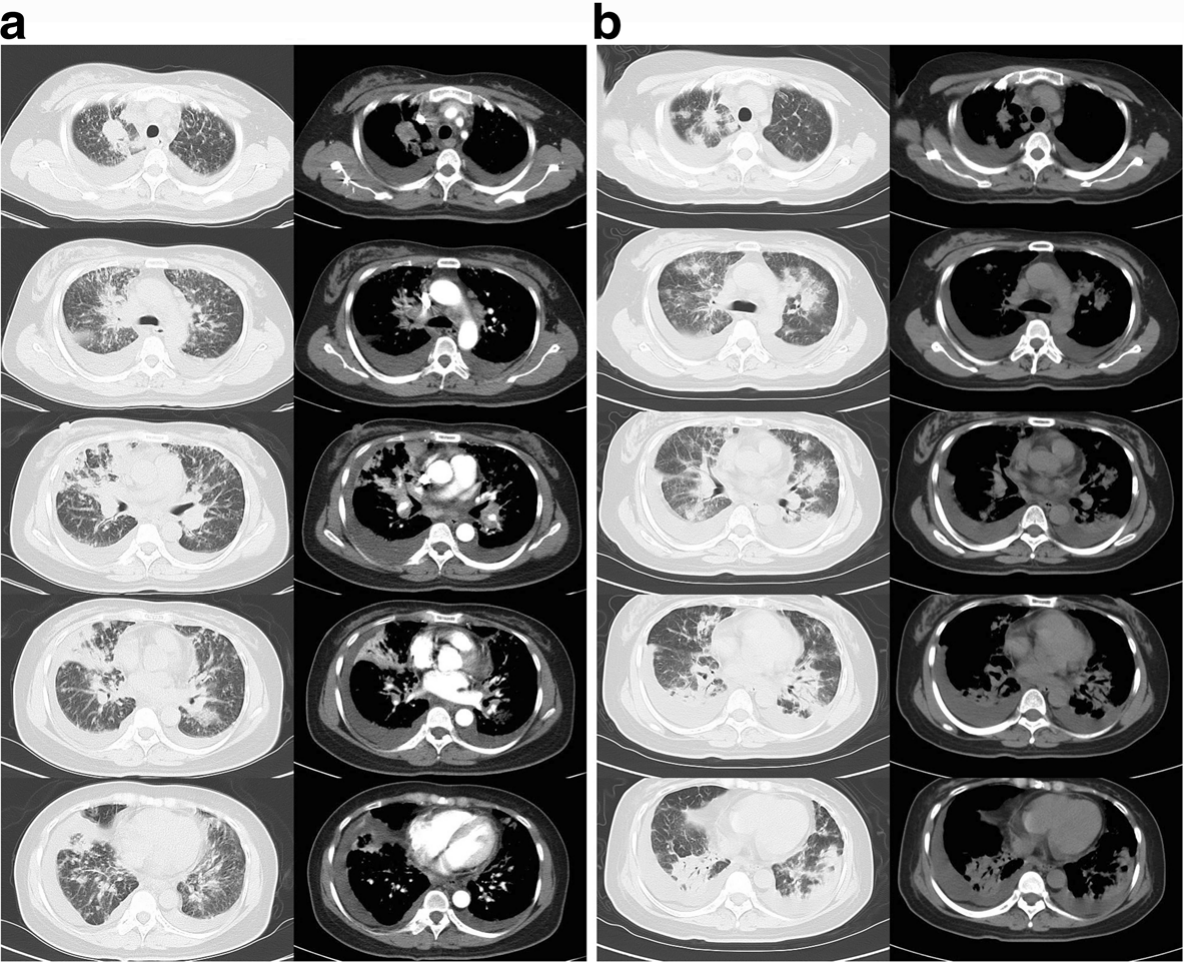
a. Prior to treatment with crizotinib, a chest CT scan revealed a large irregularly shaped mass on the upper right lobe, accompanied by multiple nodules, plaques and consolidated masses of diverse sizes randomly distributed in both lung fields. Nodular thickening of the interlobular septa and fissures, which suggested lymphangitis carcinomatosa, hilar and mediastinal lymphadenopathy, and bilateral pleural effusions, were identified as well. b Ten days after the initiation of crizotinib, chest CT revealed a significant shrinkage of intrapulmonary neoplastic lesions, lymphadenitis and lymphadenectasis but not of multiple new-onset ground-glass opacities and consolidation lesions throughout both lungs
